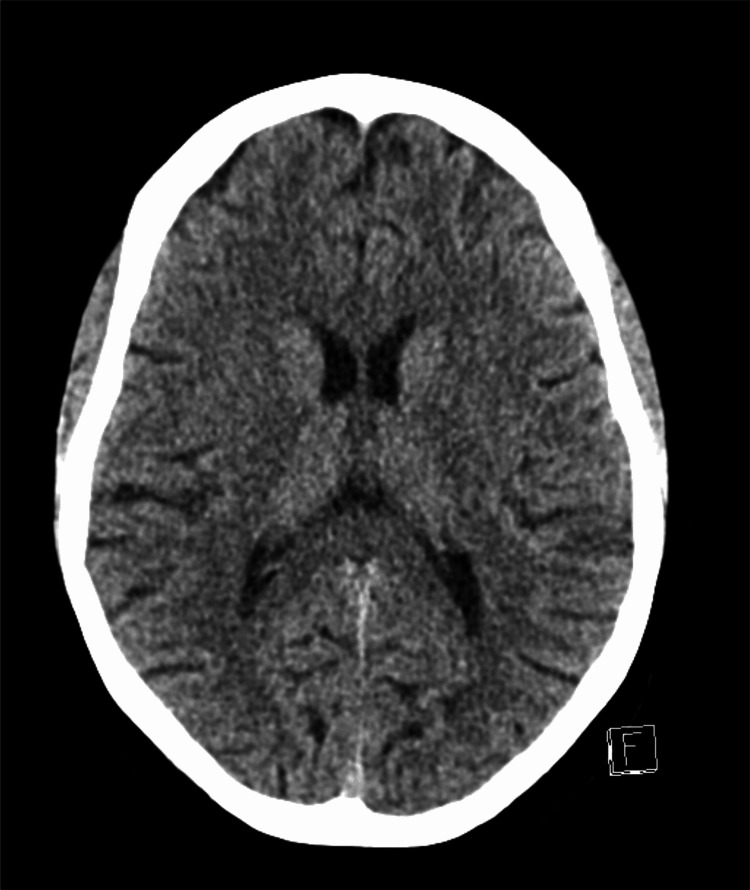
Computerized tomography (CT) scan of the brain in the axial view in a comparable view to the MRIs in Figure 2 and Figure 3, showing no acute abnormality.
